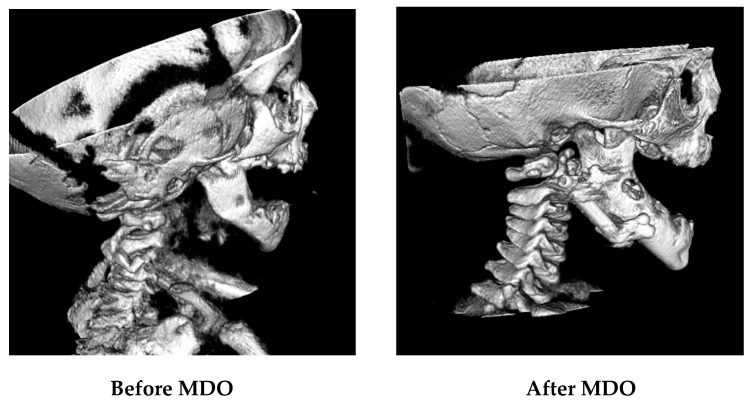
Hard tissue (bone) in CBCT images before and after MDO.
